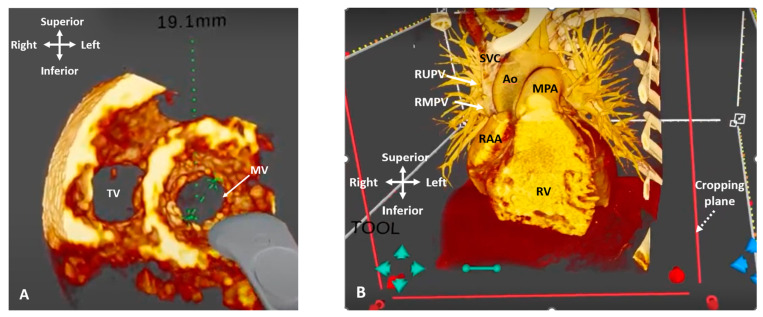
Three-dimensional rendered imaging in virtual reality. (A) 3D TOE image viewed from ventricular apex, demonstrating view of the mitral and tricuspid valves. A measurement of the mitral valve annulus has been performed using the in-built tool. (B) CT demonstrating a case of Superior Sinus Venosus ASD. The cropping plane (dashed arrow) is cropping into the volume from the anterior plane and demonstrating the entry points of the RUPV and RMPV into the SVC (arrows). Ao: Aorta; MPA: Main pulmonary artery; MV: Mitral valve; RAA: Right atrial appendage; RMPV: Right middle pulmonary vein; RUPV: Right upper pulmonary vein; RV: Right ventricle; SVC: Superior vena cava; TV: Tricuspid valve.
